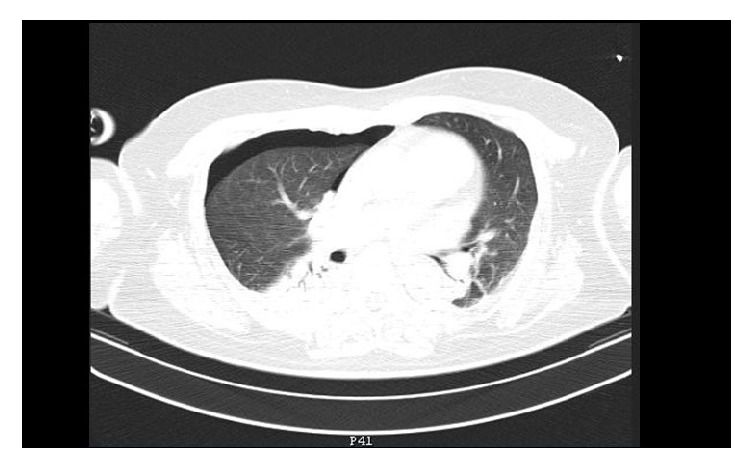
CT in the thorax showing right hemothorax and pneumothorax.
